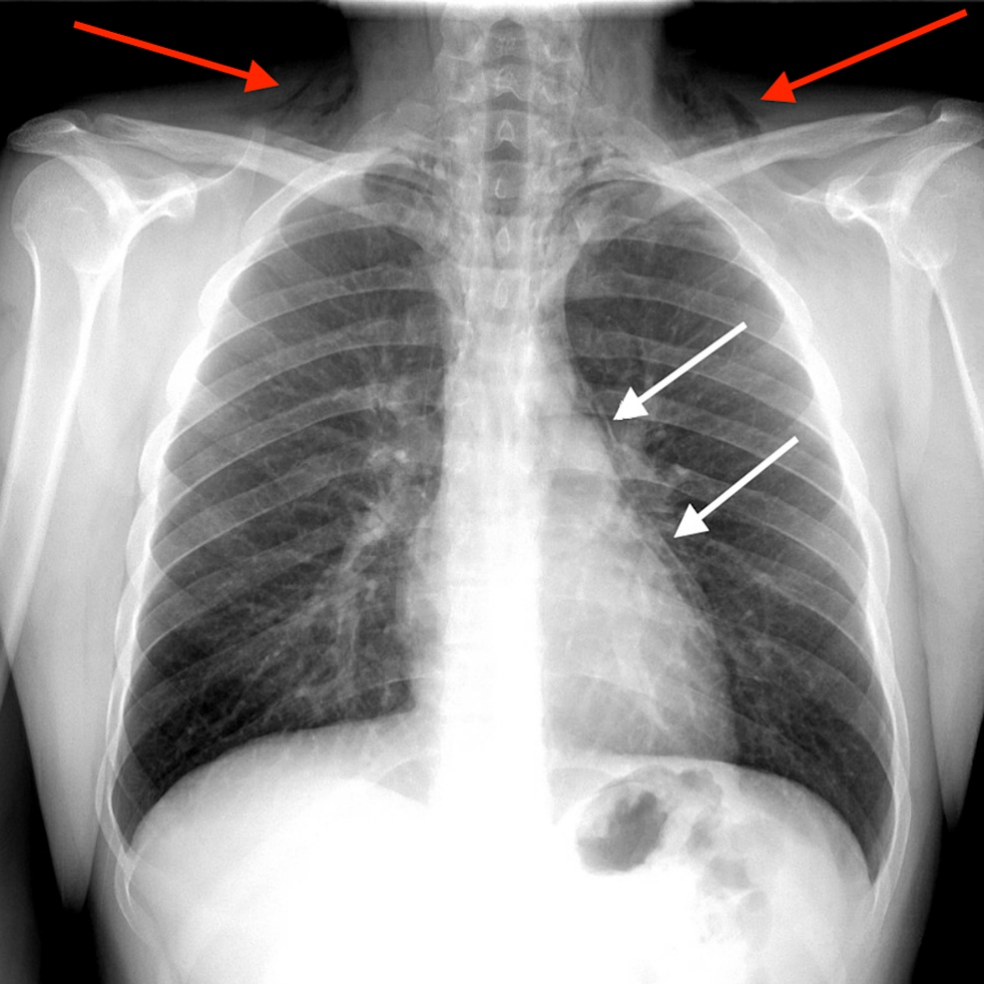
Chest x-ray showing subcutaneous emphysema (red arrows) and pneumomediastinum (white arrows)
